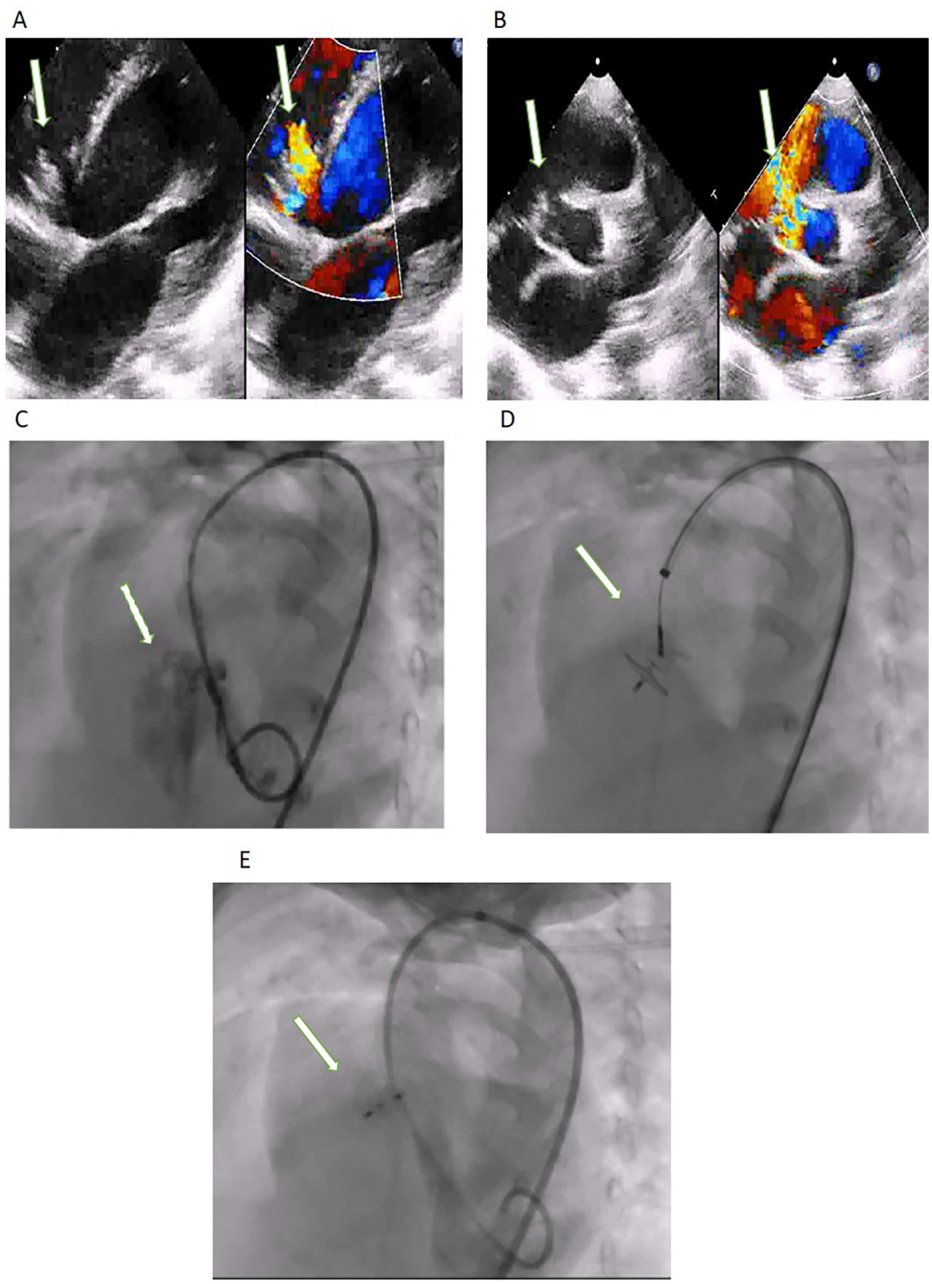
(A) TTE modified apical four-chamber with Doppler color and (B) parasternal short axis view with Doppler color showing the perimembranous VSD (arrow). (C) Left Ventricular Angiography: the arrow shows the VSD. (D) Retrograde approach showing device occluding the defect with the delivery cable attached to the LV disc. Post-device closure (E) left ventricular angiogram showing good device position (arrow) and no significant residual flow.
